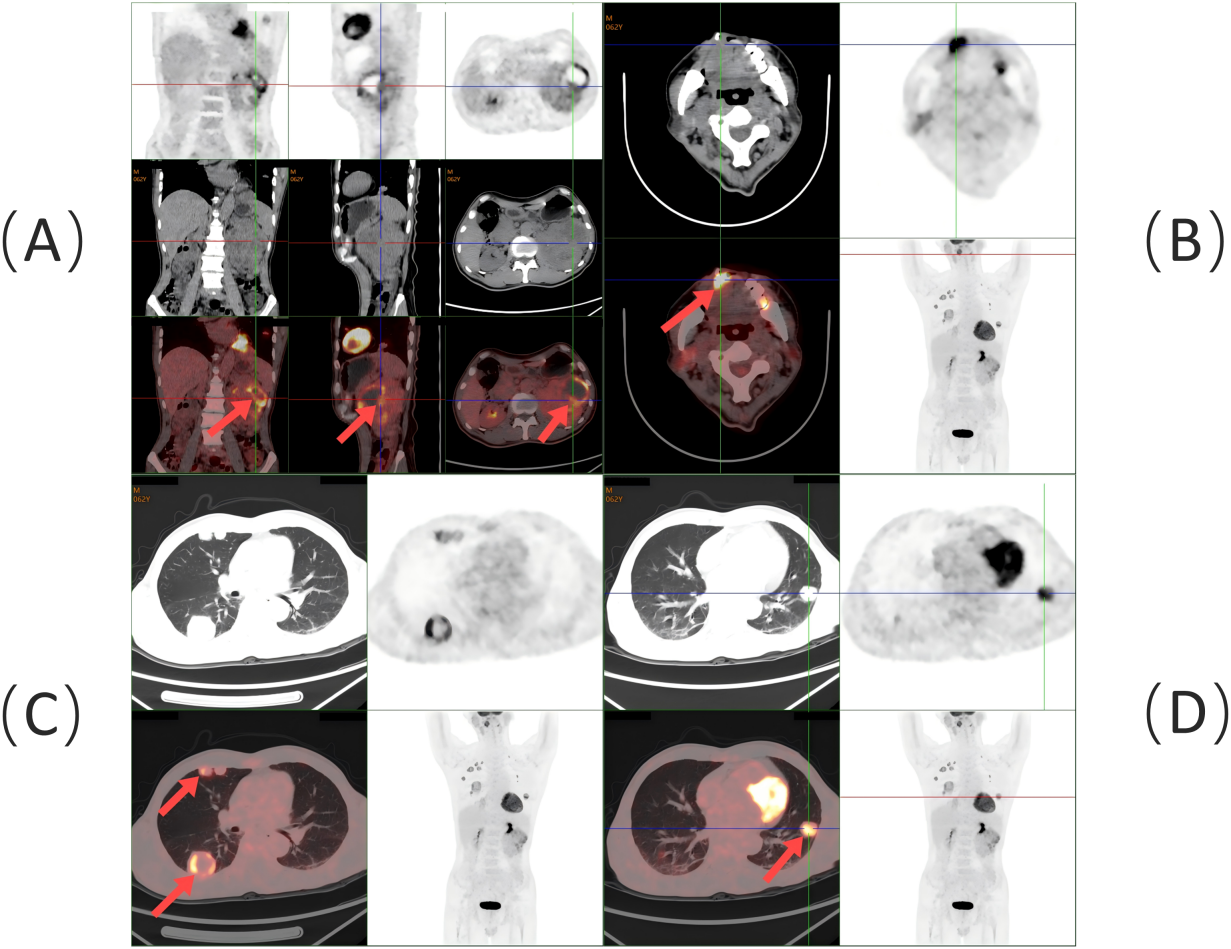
Whole-body PET/CT scan findings: (A) Soft tissue mass with ^18^F-FDG hypermetabolism in the left kidney (red arrow). (B) Soft tissue density lesion with ^18^F-FDG hypermetabolism at the right anterior border of the tongue (red arrow). (C) Multiple ^18^F-FDG hypermetabolic nodules and masses in the right lung (red arrow). (D)
